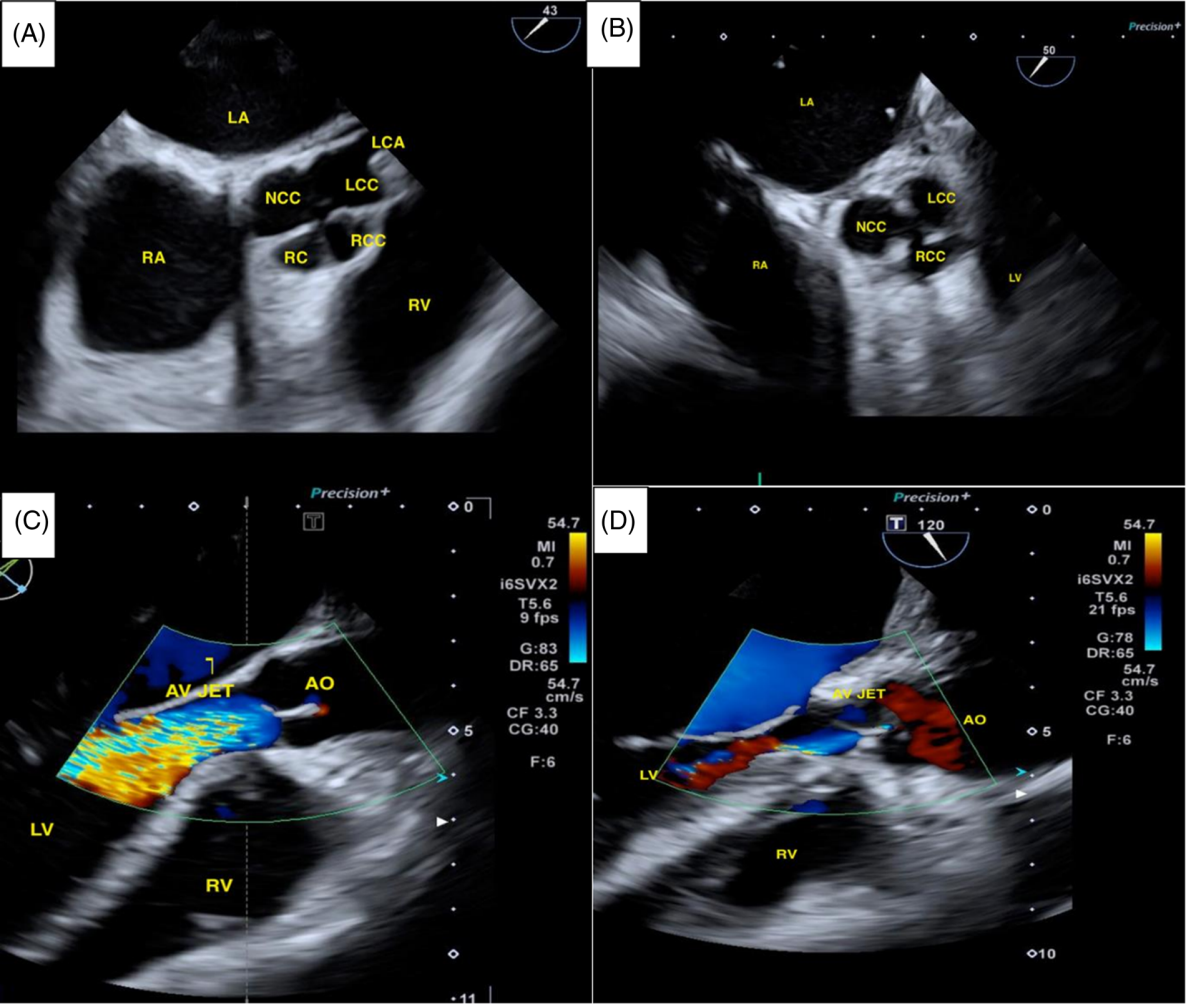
(A) Transesophageal echocardiogram showing Quadricuspid aortic valve with a rudimental cusp (RC) bond with right coronary cusp (RCC). (B) Transesophageal echocardiogram: tricuspidization of aortic valve after repair: left coronary cusp (LCC), non coronary cusp (NCC) and a unique right coronary cusp (RCC). (C) Transesophageal echocardiogram: insufficiency jet of QAV. (D) Mild residual aortic regurgitation after QAV repair. LA, left atrium; LV, left ventricle; RV, right ventricle; LCA, left coronary artery; AO, aorta; AV JET, aortic valve regurgitation doppler‐jet.
